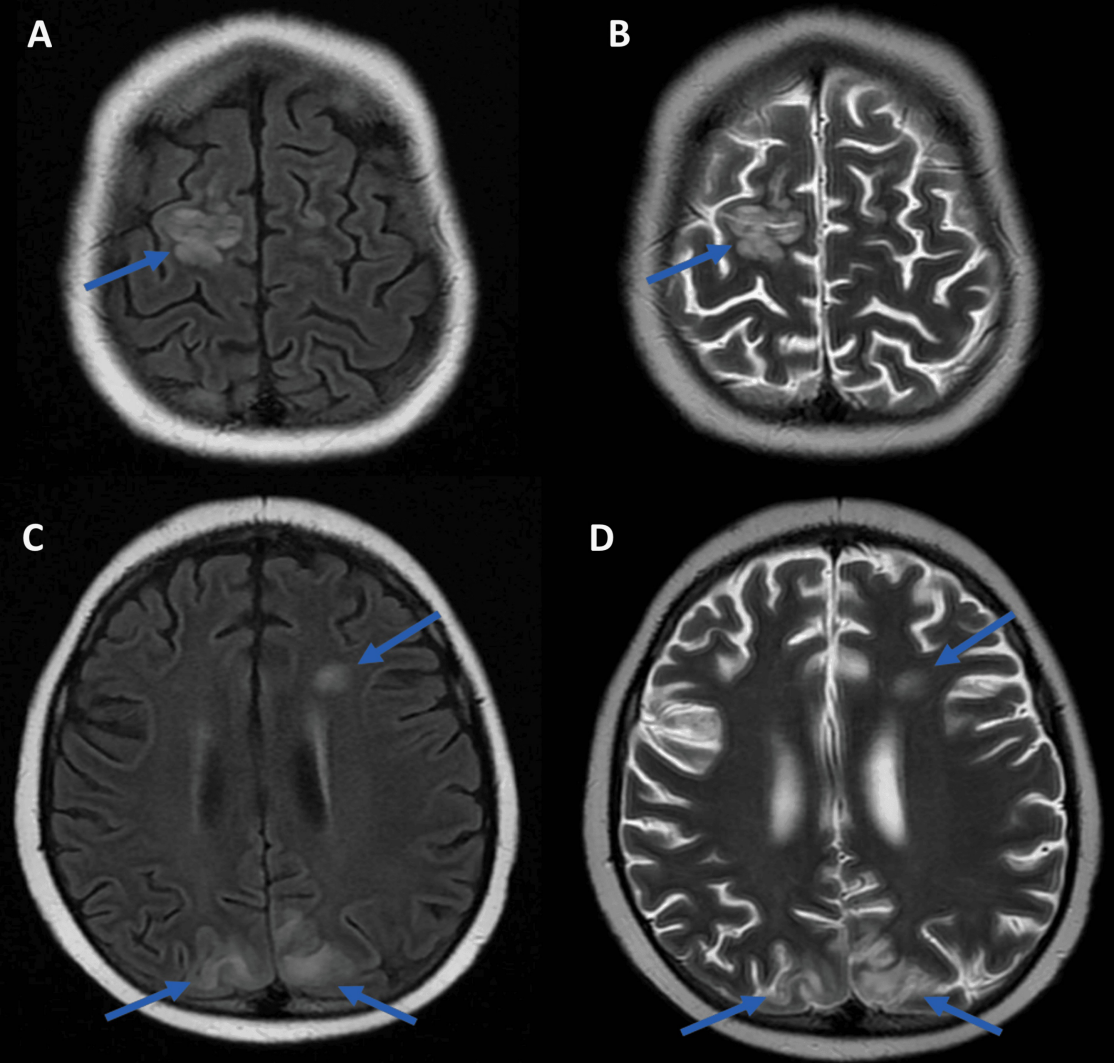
MRI images in a child with HLH and seizures FLAIR (A) and T2-weighted (B) brain MRI images in a child with HLH and seizures demonstrate multifocal hyperintense lesions (arrows in A and B) in the left frontal white matter and the right frontal (C) and biparietal cortical and subcortical regions (D) (arrows in C and D). HLH: hemophagocytic lymphohistiocytosis; FLAIR: fluid-attenuated inversion recovery
